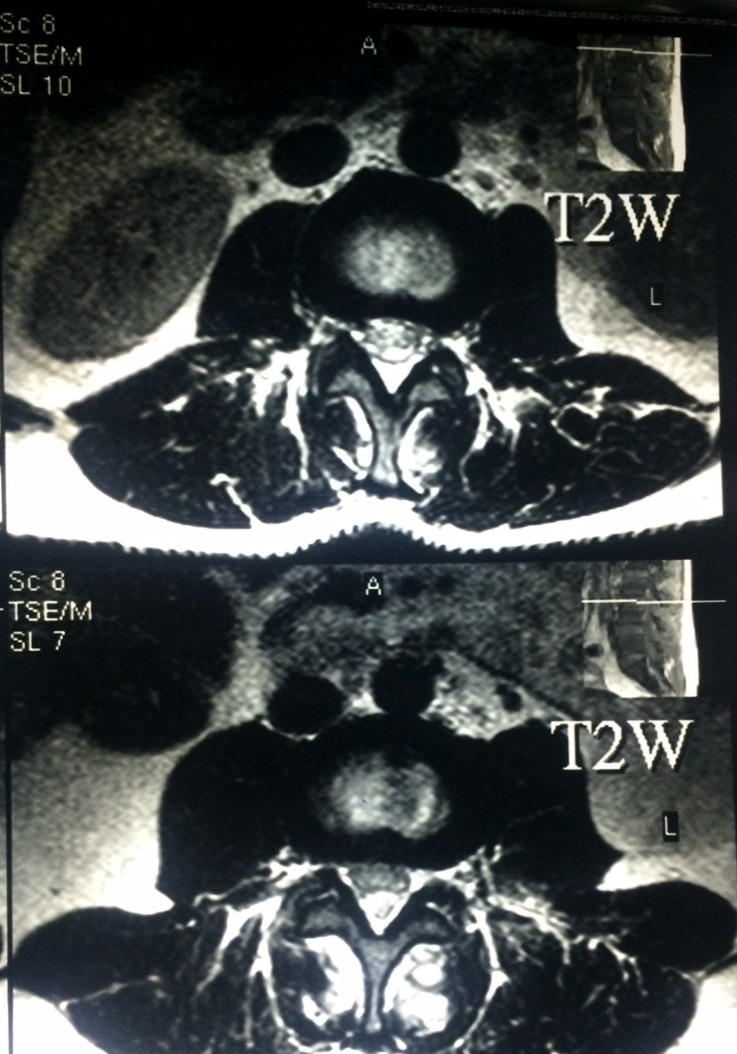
Preoperative MRI, axial section T2W  at the level of L3 & L4 show canal compression on dural by pus collection.
